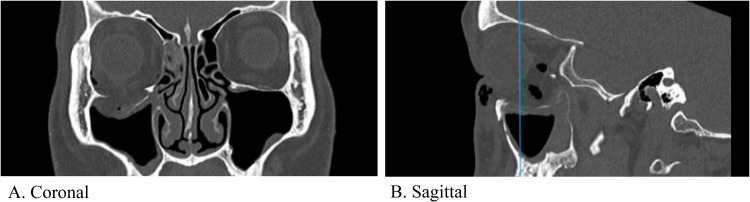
Pre-operative CT facial bones showing the orbital floor defect in coronal (A) and sagittal (B) views. Vertical line in the (B) panel reflects the corresponding slice in the coronal (A) view.
